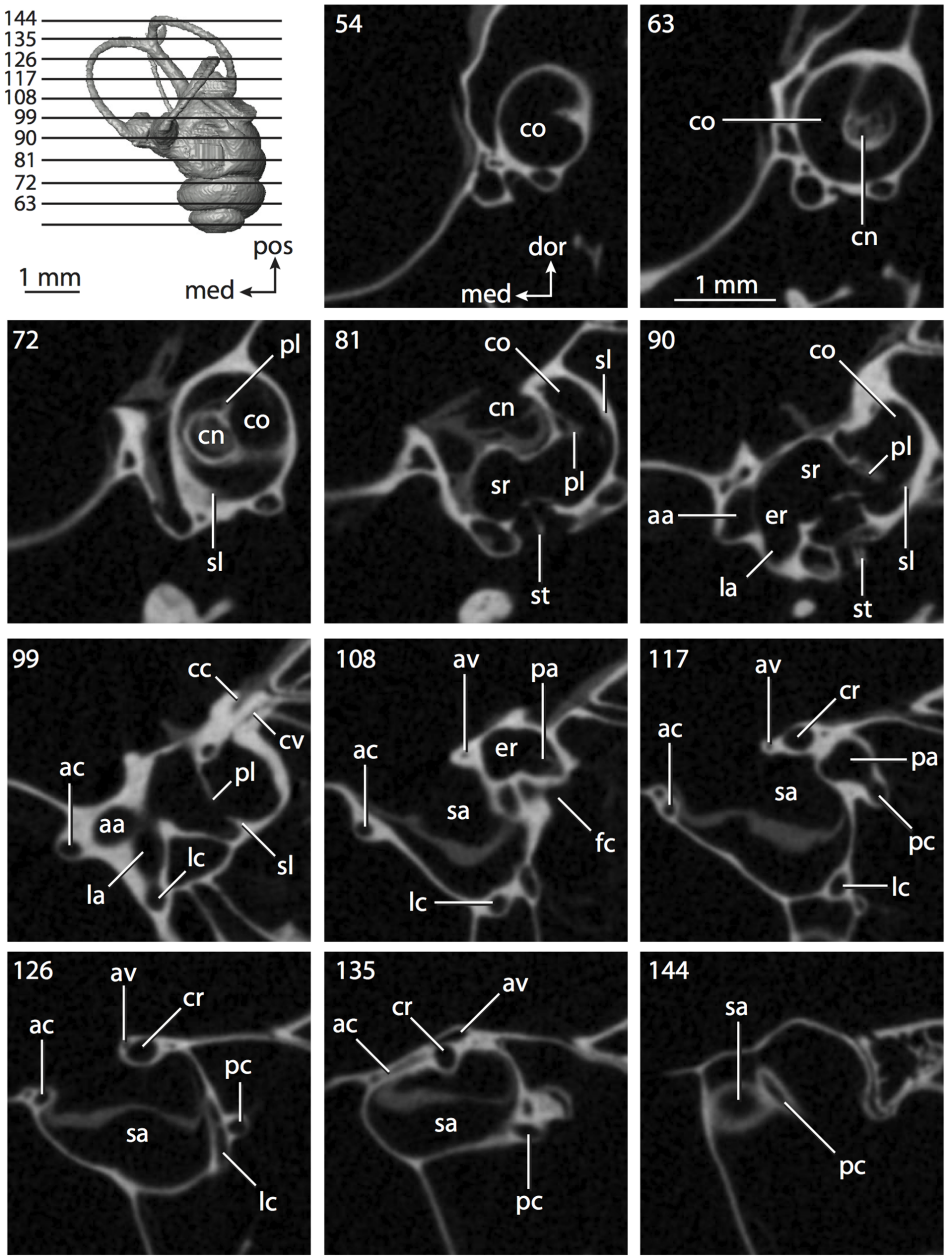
CT slices through ear region of*Macroscelides proboscideus*. Abbreviations listed at the end of the Materials and Methods section.
